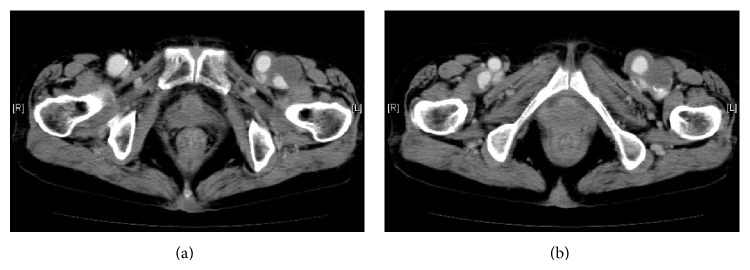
Computed tomography showed bilateral common femoral artery aneurysms (a) and bilateral profunda femoris artery aneurysms (b).
